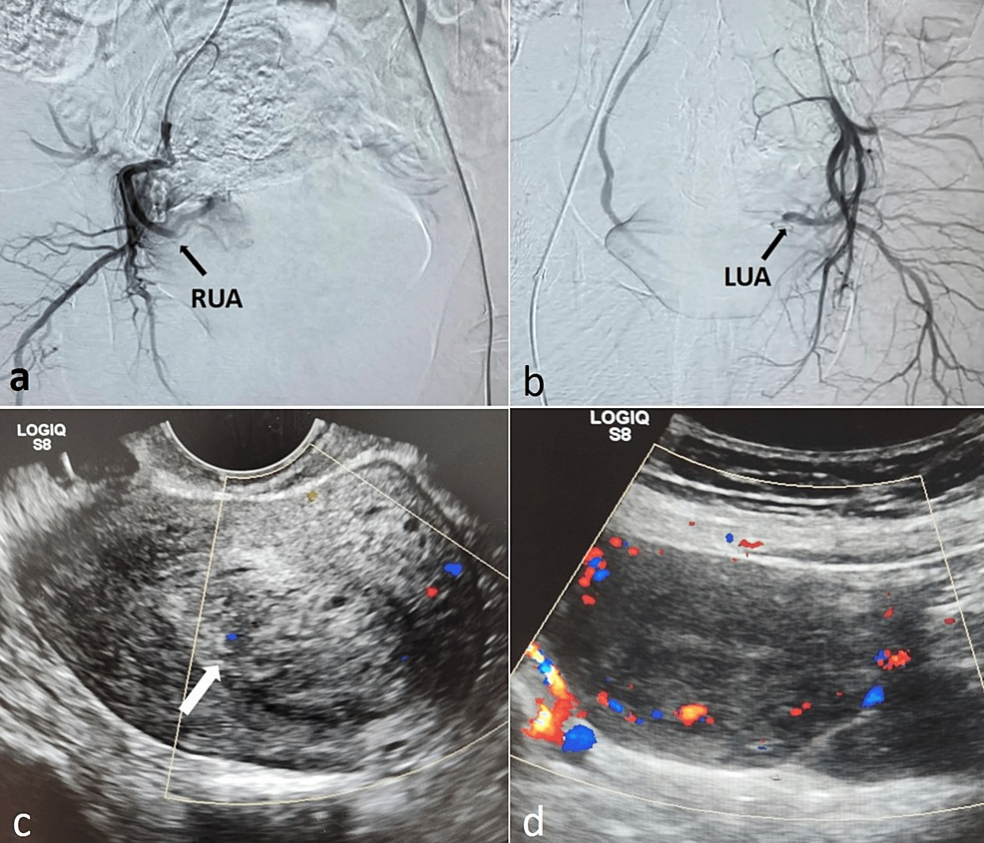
Post-embolization DSA and US images of the patient with AVM (a, b) Post-embolization DSA images showing non-opacification of the uterine AVM after embolization with NBCA/glue. Post-UAE: (c) Transvaginal US image on day 1 of intervention showing a bulky uterus with a heterogeneous complex-echoic mass within the endometrial cavity (white arrow); however, no abnormal vascularity was detected in the uterine wall on color Doppler. (d) Transabdominal US image after two months showing a normal-sized uterus with no endometrial mass or abnormal vascularity. AVM, arteriovenous malformation; DSA, digital subtraction angiography; LUA, left uterine artery; NBCA, n-butyl-2-cyanoacrylate; RUA, right uterine artery; UAE, uterine artery embolization; US, ultrasound
